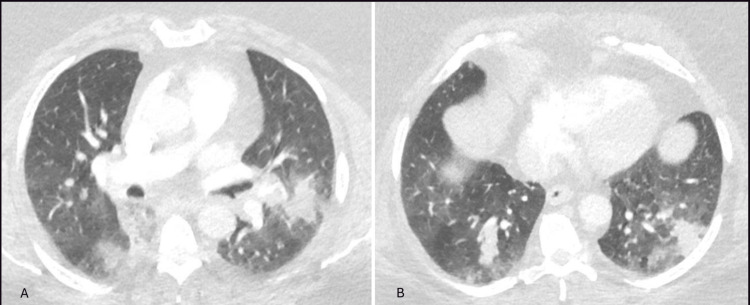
Axial view of chest CT scan (lung window) showing worsening bilateral opacities (as compared to the CT scan on admission). Panel A showing new left lower lobe dense opacities. Panel B showing persistent bilateral lower lobe opacities.
